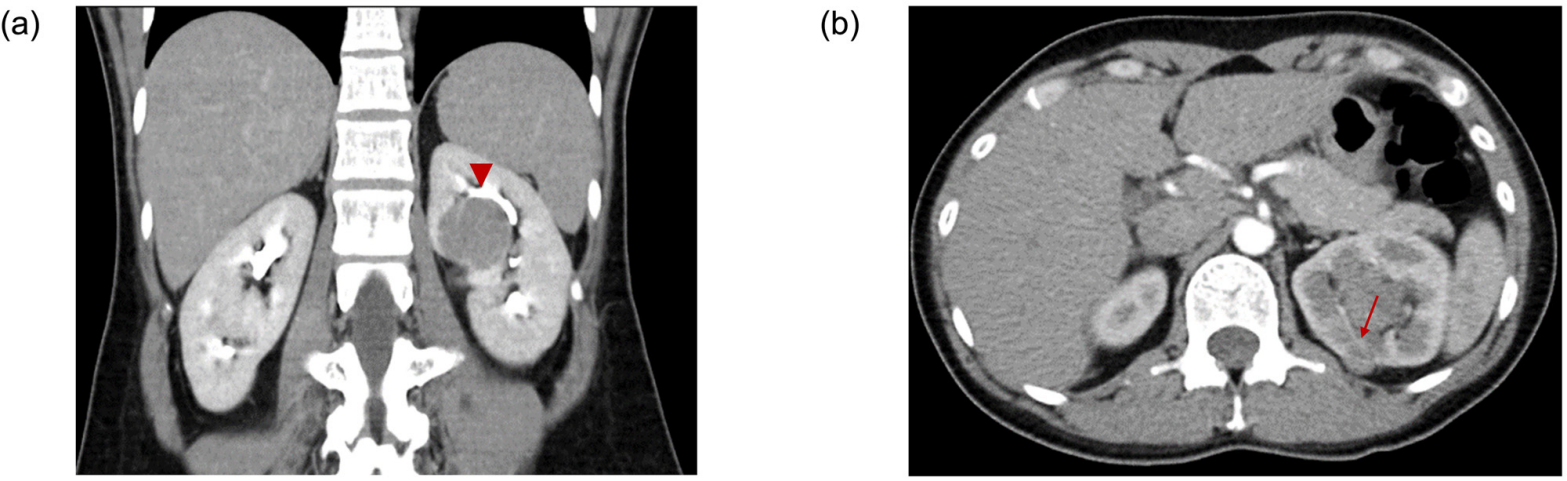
Abdominal CT revealed (a) the main endophytic tumor (arrow head) in the dorsal interpolar region of left kidney and (b) another exophytic tumor (arrow).
